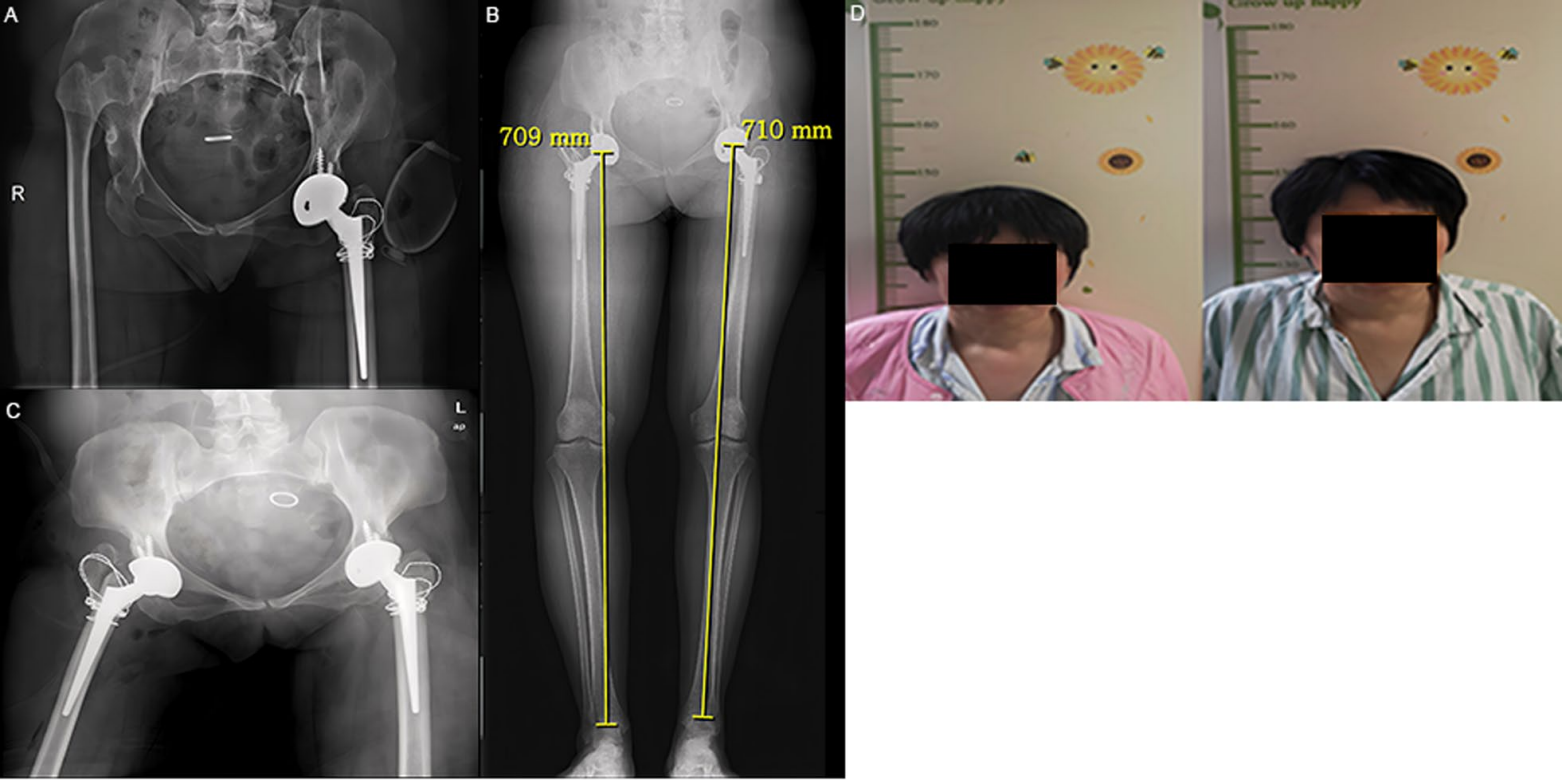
Three months later, the preoperative X-ray of the right THA was performed at the second stage. A The X-ray image after the first operation; B On the third day after the second stage of right THA; C The full-length X-ray of both lower limbs in a standing position showed that the length difference of both limbs was 0.1 cm; D The height was measured on the third day after the second operation. Compared with that before the operation, the height was about 7.5 cm longer, and the LLD was 0.1 cm
